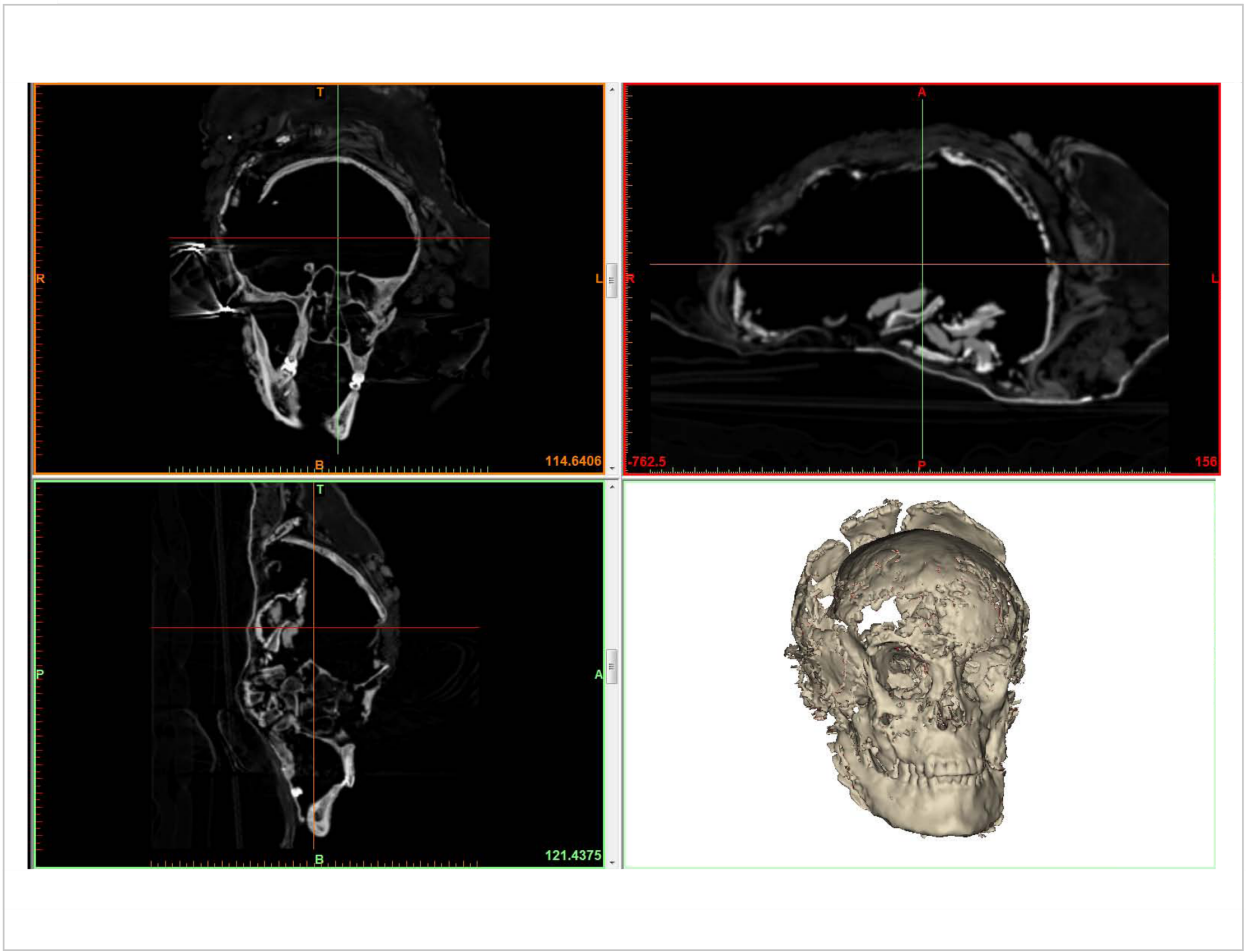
CT images (axial, coronal, and sagittal views) and 3D visualization of the skull.
